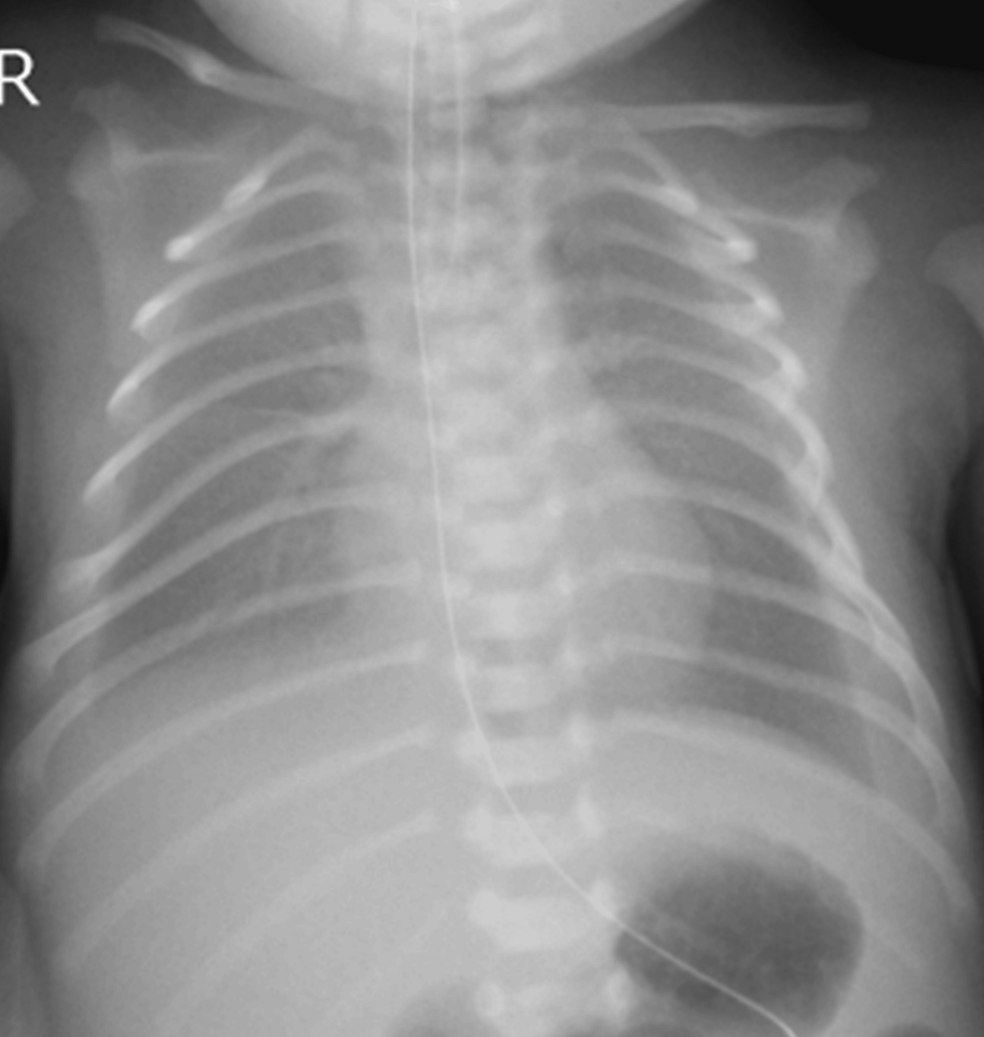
Chest radiograph on admission to the neonatal intensive care unit Bilateral pleural effusions with right interlobar pleural effusion are seen.
